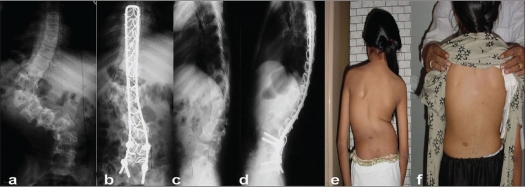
Preoperative and post operative x-rays antero posterior and lateral views (a-d) shows a novel concept of sacral fixation using pedicular screws in L4-S1, fixed to the split arms of the rectangle, in severe dystrophic curve in neurofibromatosis. Preoperative and post operative clinical photographs (e,f) shows significant correction in both planes and good spinal function at five years follow up
